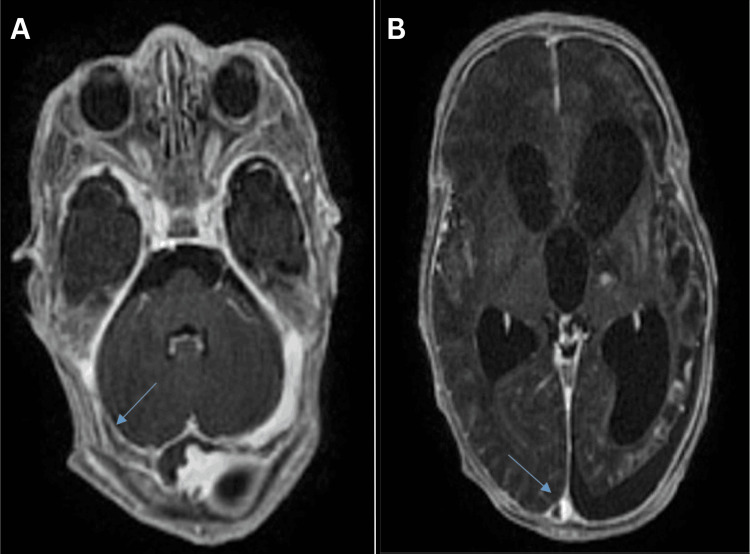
(A) Brain MRI in axial T1 sequence with gadolinium injection showing persistence of endoluminal material within the lateral sinuses (blue arrow). (B) Brain MRI in axial T1 sequence with gadolinium injection showing persistence of endoluminal material within the superior sagittal sinus (blue arrow). MRI: magnetic resonance imaging
